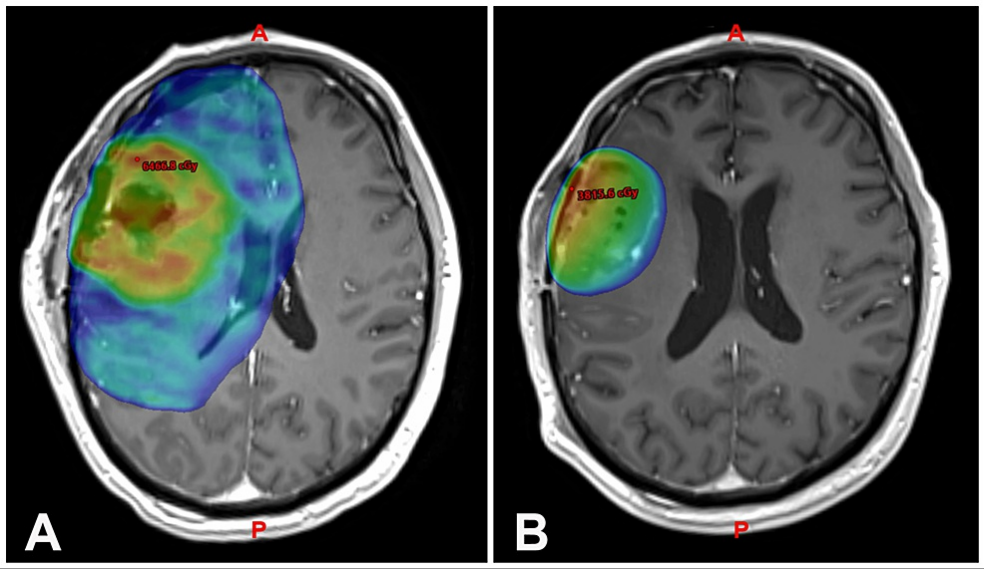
ReFSRT plan demonstrating reduced target volumes on axial MRI T1+1 gadolinium-enhanced sequence (A) Initial target volume for 30 fraction irradiation and (B) ReFSRT target volume
